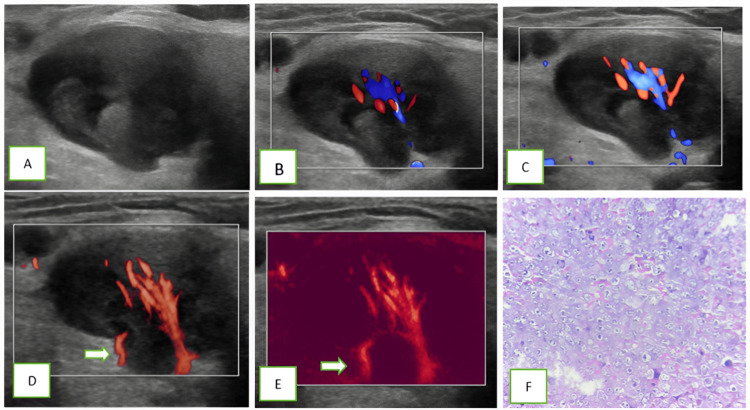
Metastatic deposits from poorly differentiated epithelial malignancy. An elderly chronic smoker presented with breathlessness, abdominal pain, and cervical lymphadenopathy for 6-8 months. (A) Gray-scale ultrasound (US) image showing an enlarged cervical lymph node with loss of normal hilar architecture and cortex. (B, C) Color Doppler and power Doppler, respectively, revealed hilar vascularity. (E, F) MVI (color) and MVI (power) modes, respectively, demonstrated peripheral neovascularization (white arrows)-likely suggesting metastatic malignant lymphadenopathy. (F) FNAC shows clusters of atypical malignant epithelial cells with pleomorphic hyperchromatic nuclei, consistent with metastatic deposits from poorly differentiated epithelial malignancy. MVI: microvascular imaging; FNAC: fine-needle aspiration cytology
